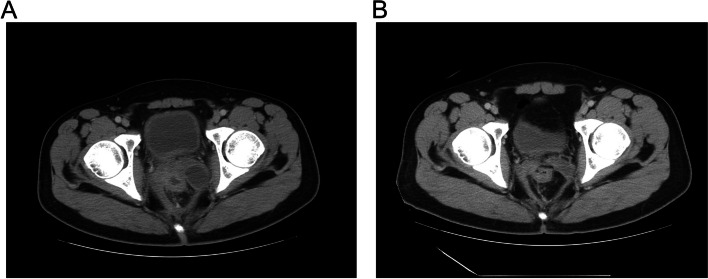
Follow-up condition of the patient. A The tumor recurred during chemotherapy. B The recent computed tomography (CT) scan showed that the tumor had disappeared after the patient received 4 cycles of immunotherapy
